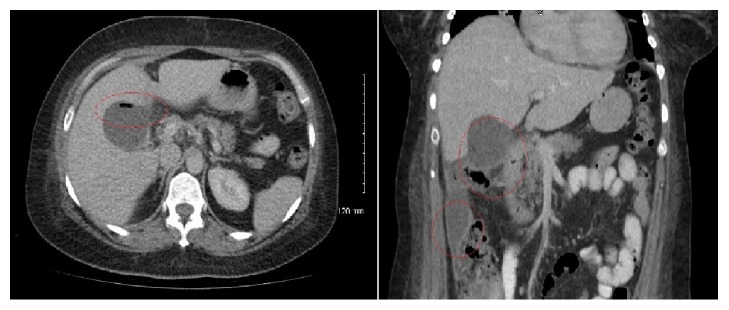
CT abdomen pelvis day 6: air within a distended gall bladder with persistent pericholecystic fat stranding, more marked than on prior imaging and extending to involve the hepatic flexure. New, peripherally enhancing fluid collection extending the inferior tip of the liver along the right paracolic gutter; appearances are suggestive of complicated cholecystitis with a gallbladder perforation.
